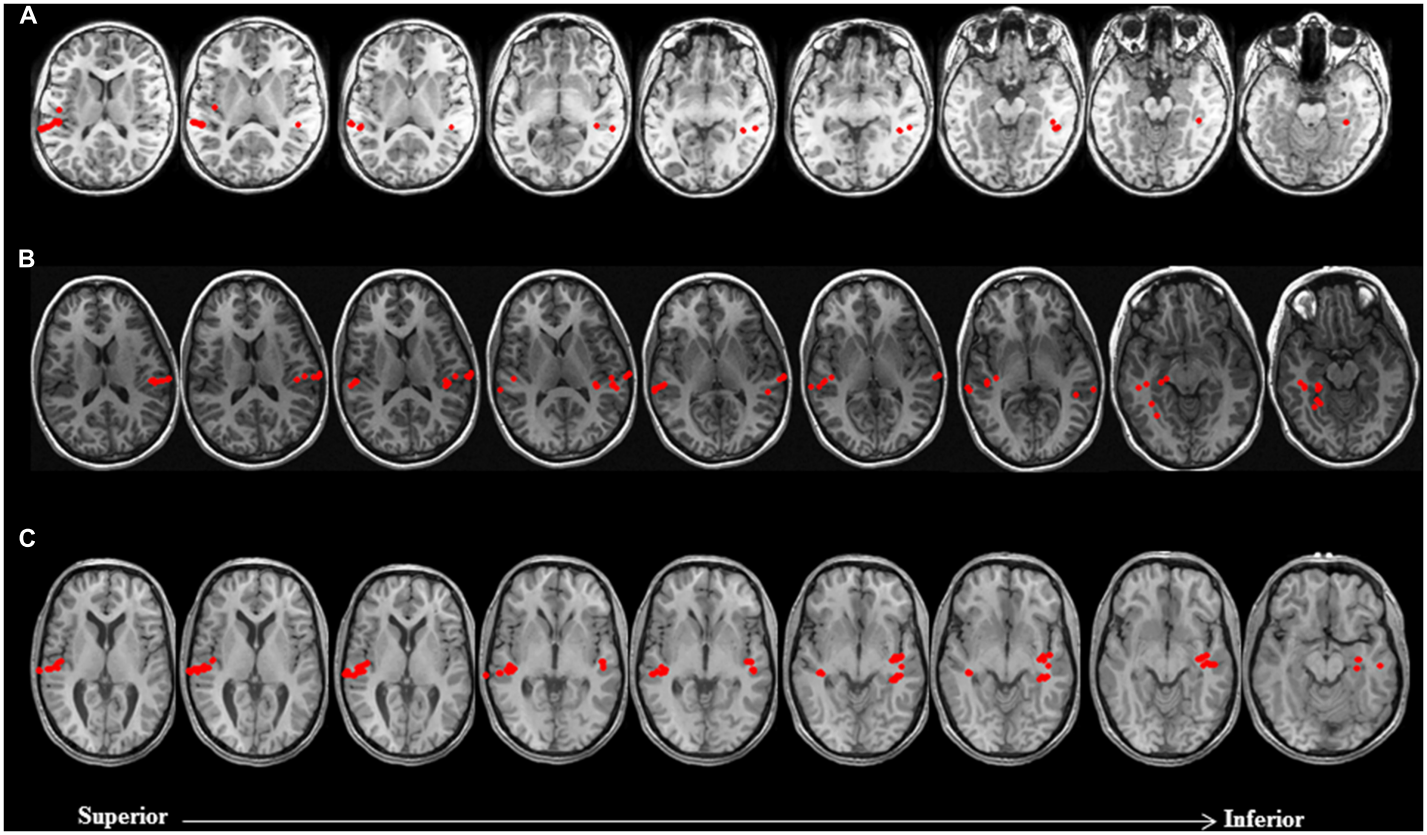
Discordance between laterality judgments based on LI estimates and visual inspection of brain activation profiles in three non-sedated patients. Language activity (dipolar) sources are represented as solid red circles projected onto the patients’ MRI, displayed in radiological convention. (A) 9 year-old female with symptomatic partial epilepsy of left temporal lobe origin was deemed to be right dominant for receptive language based on LI (-0.54). Visual inspection of brain activation profiles revealed despite a greater number of activity sources concentrated in the auditory cortex of the right hemisphere, posterior and mesial temporal engagement in the left hemisphere suggested that patient was left dominant for receptive language. (B) 14 year-old female with cryptogenic partial epilepsy of left temporal lobe origin was deemed to be left dominant for receptive language based on LI (0.43). Visual inspection of brain activation profiles revealed despite a greater number of activity sources concentrated in the auditory cortex of the left hemisphere, lateral and mesial temporal engagement in the right hemisphere suggested that patient was right dominant for receptive language. (C) 17 year-old male with idiopathic generalized seizures was deemed to have bilateral representation for receptive language based on LI (0). Visual inspection of brain activation profiles revealed despite an equal number of activity sources bilaterally, posterior middle and mesial temporal engagement in the left hemisphere suggested that patient was left dominant for receptive language.
